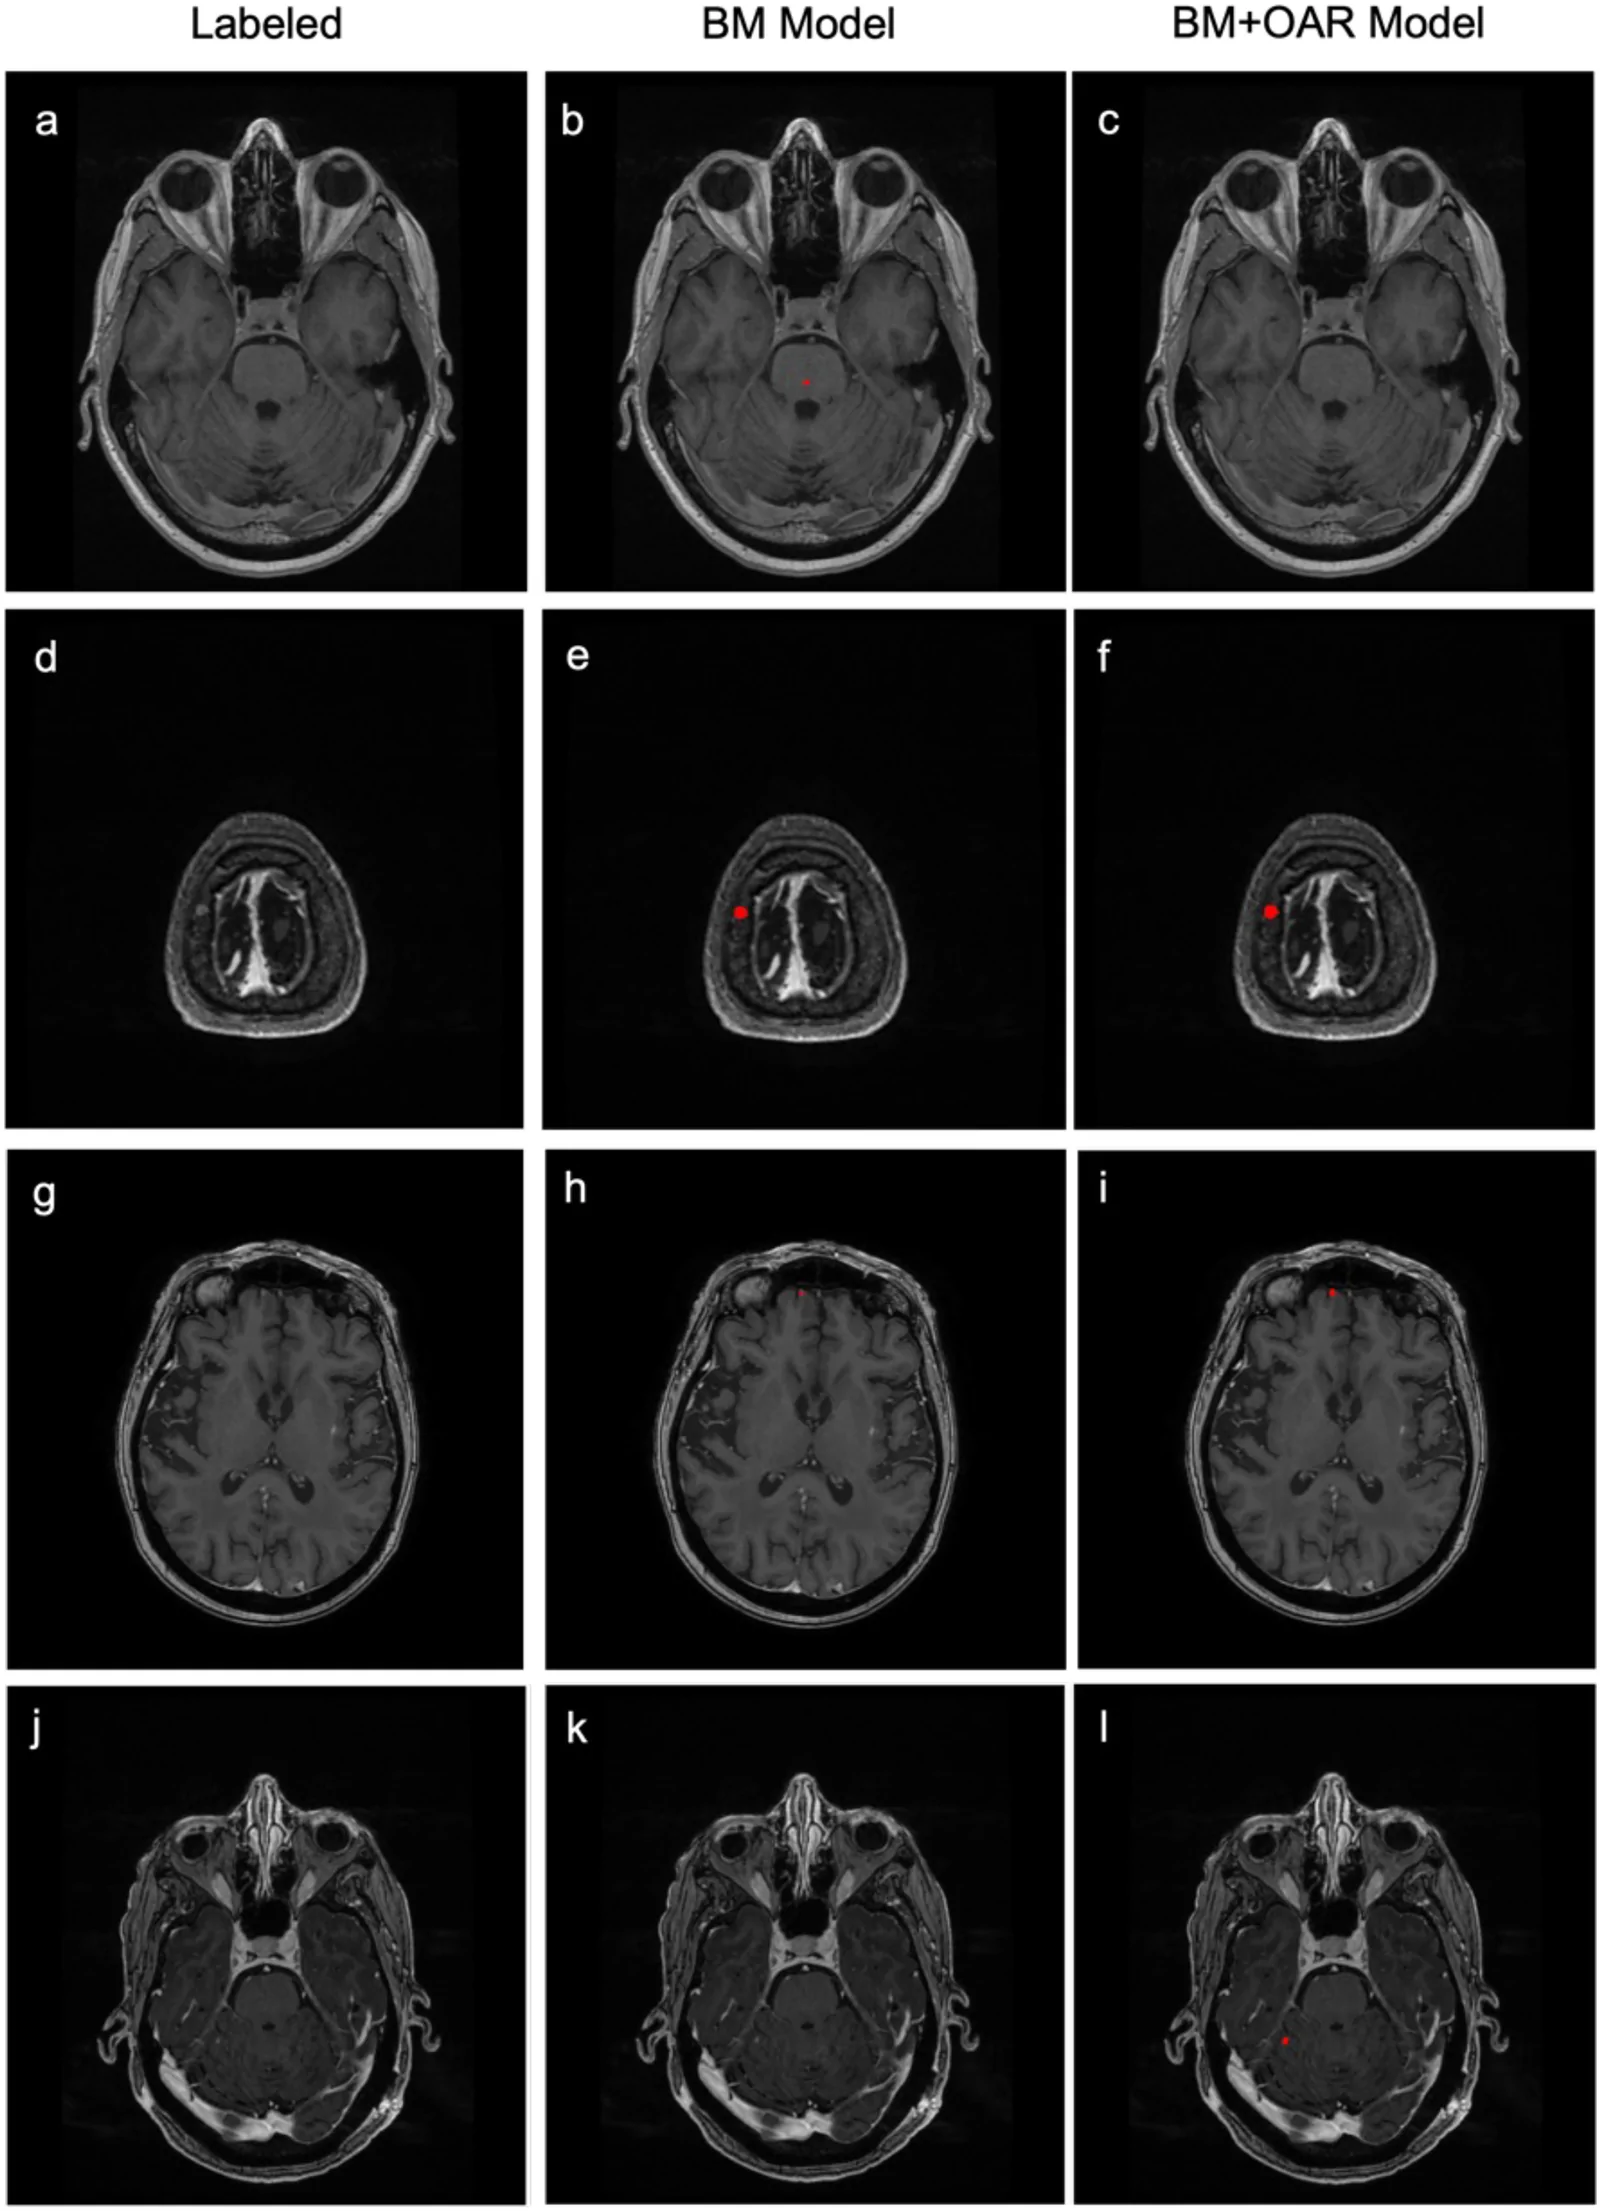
Representative false-positive identifications. (a-c) A region in the brainstem spuriously labeled as a lesion by the BM model, (d-f) a lesion in the skull mislabeled as a brain metastasis by both BM and BM+OAR models, (g-i) an unlabeled lesion identified by the BM and BM+OAR models which grew on a subsequent MRI, (a-c) an unlabeled lesion identified by the BM+OAR but not the BM model which grew on a subsequent MRI. *Abbreviations*: BM = brain metastases; MRI = magnetic resonance imaging; OAR = organ at risk.
